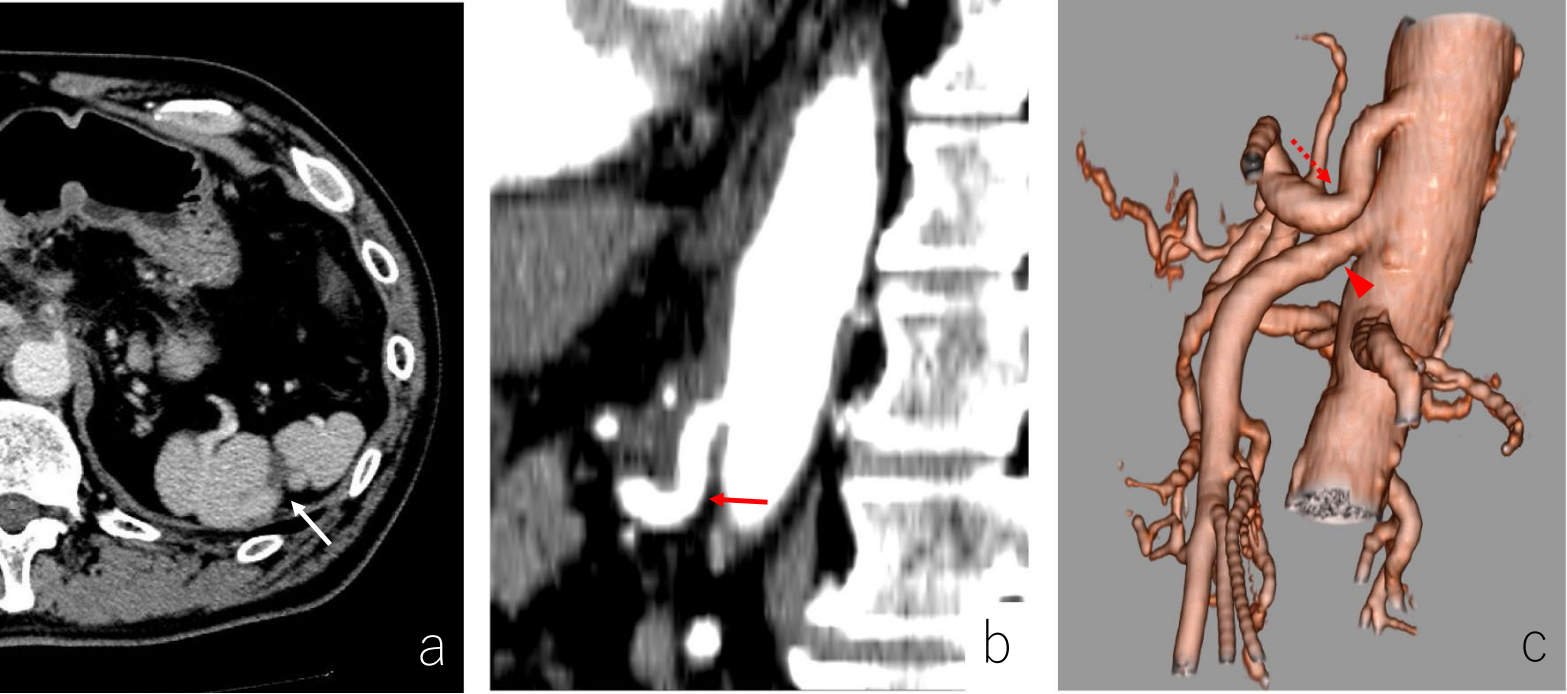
Postoperative enhanced CT. a A month after surgery, enhanced CT reveals that the poorly perfused region of the spleen is markedly reduced (white arrow). b Sagittal image of enhanced CT shows improvement of stenosis of the celiac arterial root (red arrow). c Three-dimensional reconstructed image indicates improvement of stenosis at the root of the celiac artery (dotted red arrow) and the superior mesenteric artery (red arrowhead)
